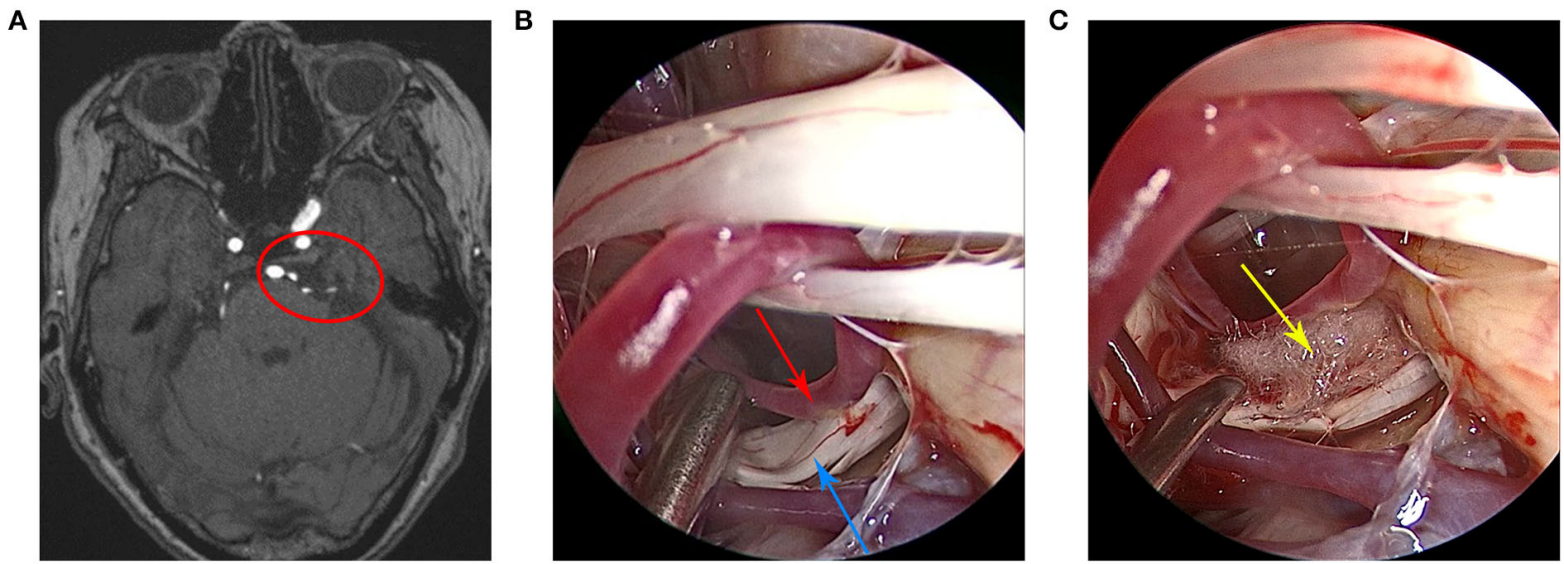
(A) Preoperative head magnetic resonance imaging of the patient. (B) The trigeminal nerve and the anterior cerebellar artery were clearly visible under the endoscopic view. (C) The Teflon pad was placed between the trigeminal nerve and the anterior cerebellar artery under the endoscopic view. The red circle indicates that the trigeminal nerve was compressed by the anterior cerebellar artery, the red arrow indicates the anterior cerebellar artery, the blue arrow indicates the trigeminal nerve, and the yellow arrow indicates the Teflon pad.
